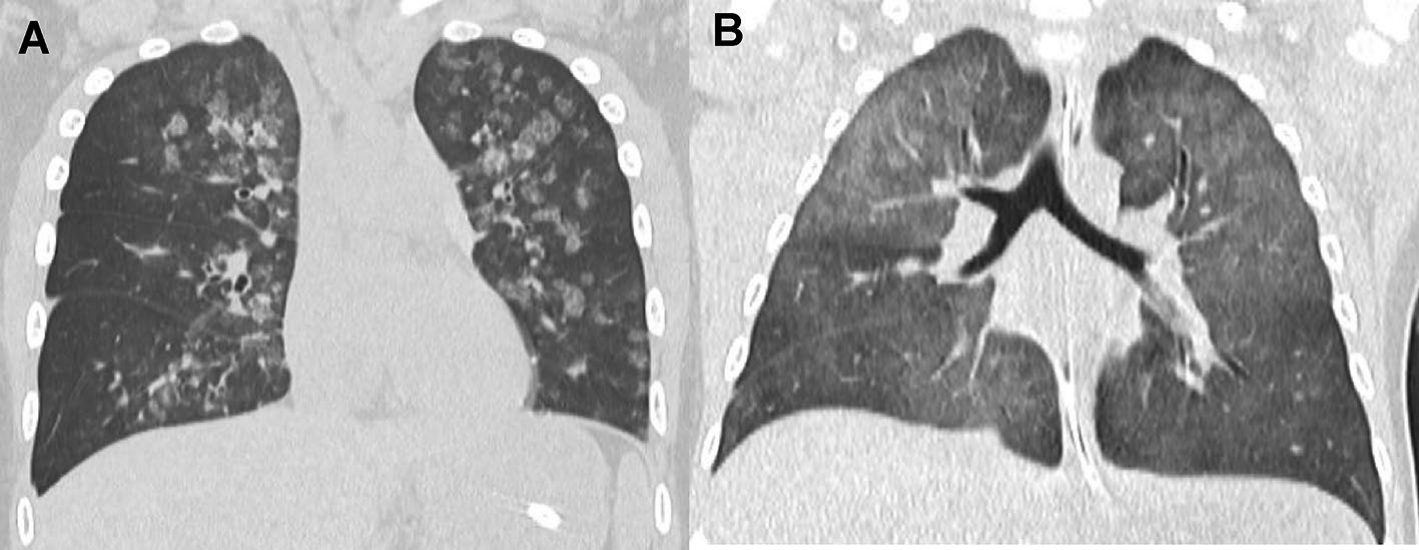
Negative-pressure pulmonary edema (2 cases). A An 8-year-old boy after choking. CT sowed ill-defined centrilobular nodules and interlobular septal thickening mainly in the parahilar region. B A 9-month-old infant after drowning. CT showed upper lung-dominant diffuse GGOs with subpleural sparing
